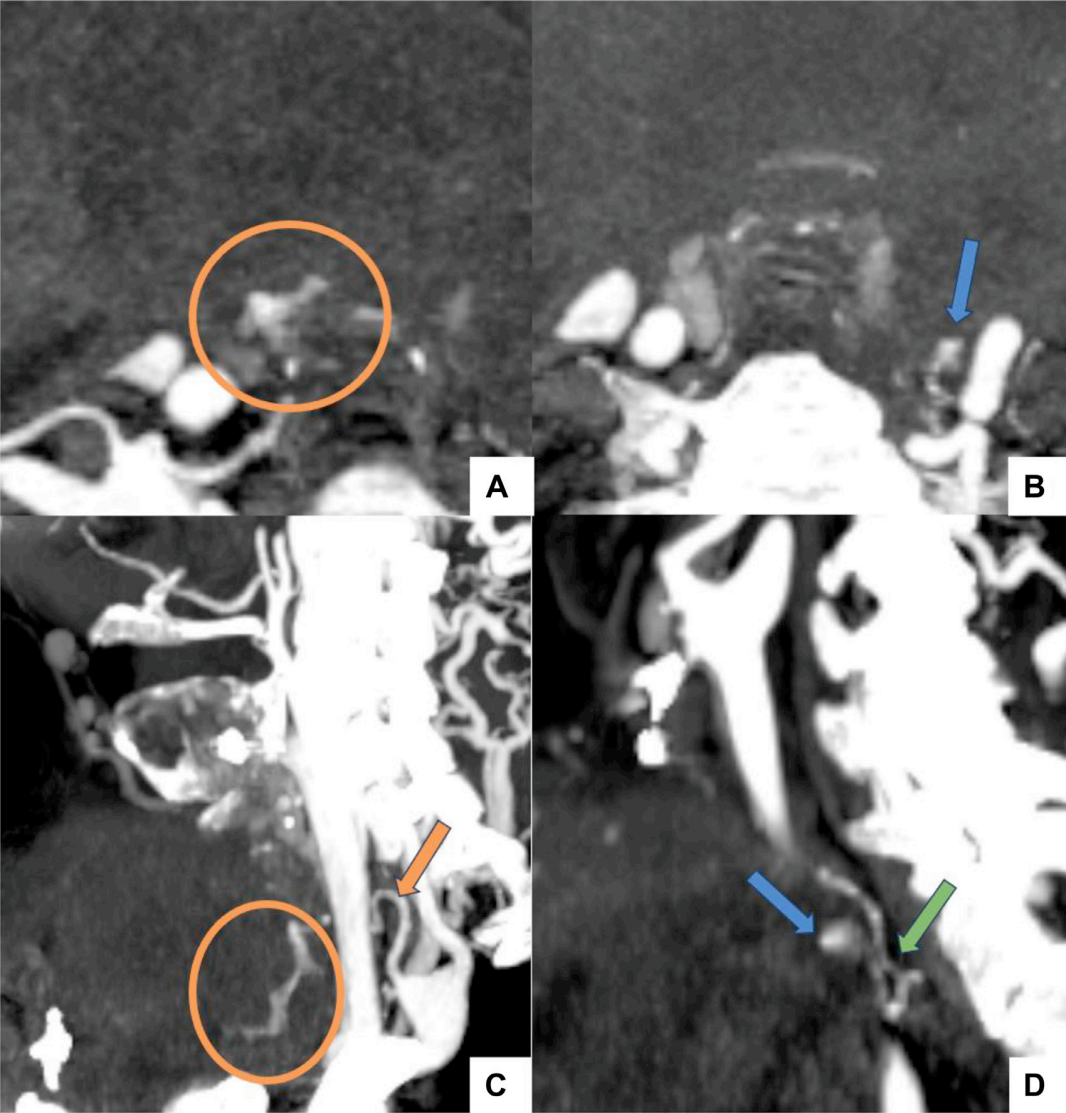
Axial (A, B) and Oblique Sagittal (C, D) cervical CTA images: Large cervical hematoma with an arterial blush (orange circle) arising from the right ITA (orange arrow), confirming an active bleeding (A, C). A small pseudoaneurysm (blue arrow) arising from the left ITA (green arrow) (B, D).
